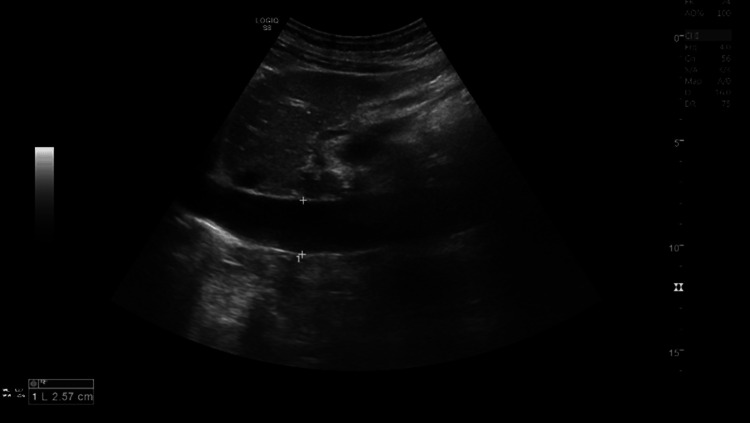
Abdominal ultrasound showing mild hepatomegaly
